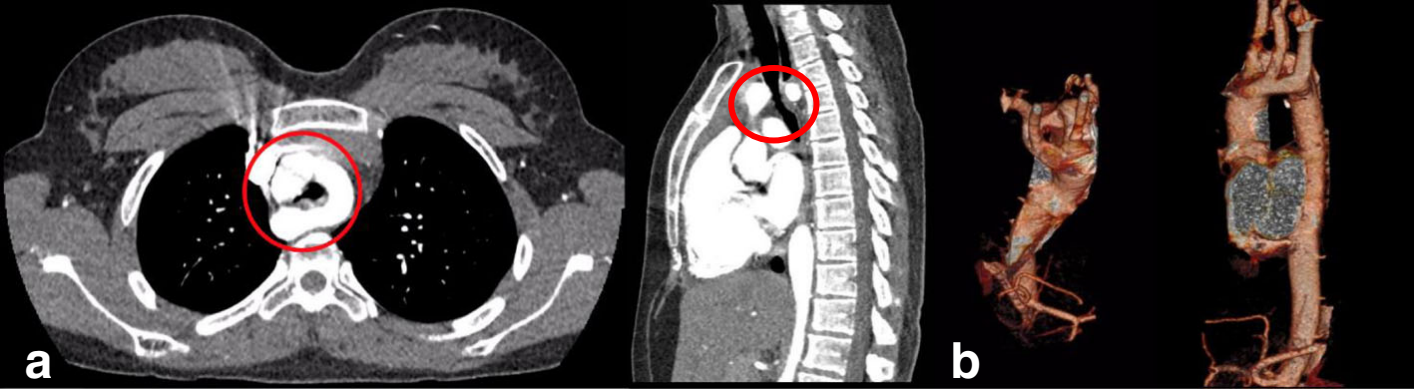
a CT scan with contrast medium highlighting the presence of a complete Double Aortic Arch (axial and sagittal reconstructions). Red circles indicate the 2 arches surrounding the trachea and esophagus. b Arterial phase CT: 3D reconstructions of the aorta, showing the DAA
